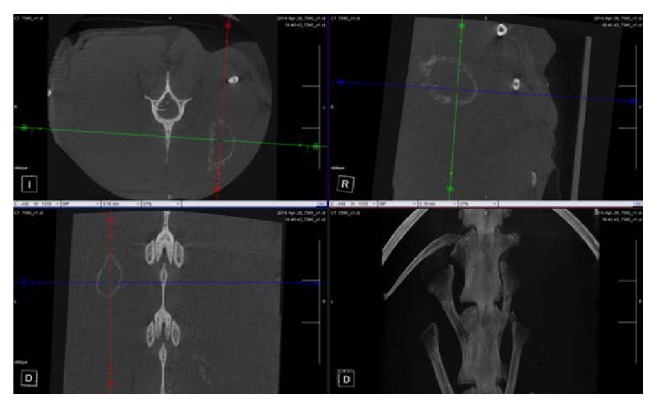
Micro CT appearance of material*. Confirmation of radiographic findings on *μ*CT demonstrating a “wispy” halo appearance at the implantation sites.
